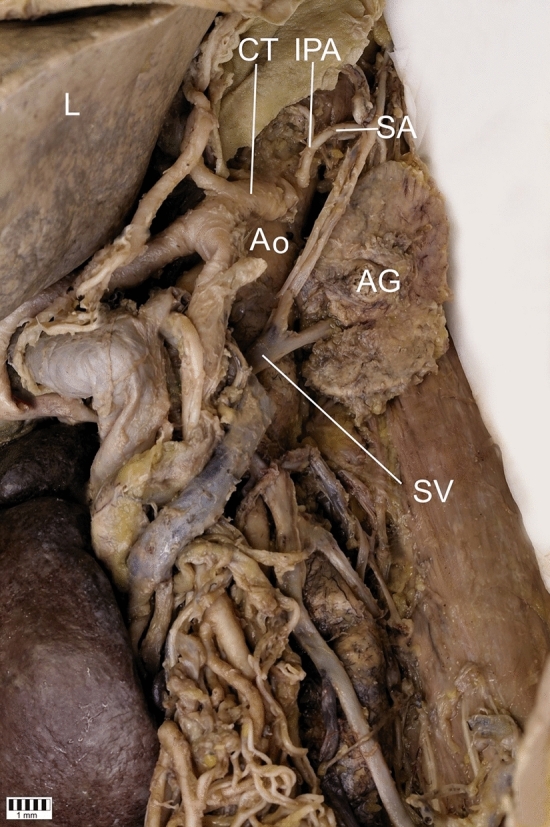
Adrenal gland in the left renal fossa. *AG* Adrenal gland, *Ao* Abdominal Aorta, *CT* Celiac Trunk, *IPA* Inferior Phrenic Artery, *L* Liver, *SA* Suprarenal Artery, *SV* Suprarenal Vein, *RV* Renal Vein
